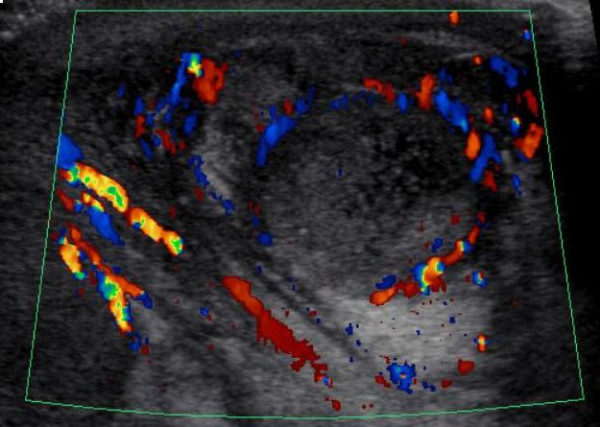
Ultrasound scan at case presentation. Doppler ultrasound scan image showing focal heterogeneity with decreased vascularity in the left upper testicle consistent with a small laceration, subscapular hematoma and intraparenchymal hematoma.
